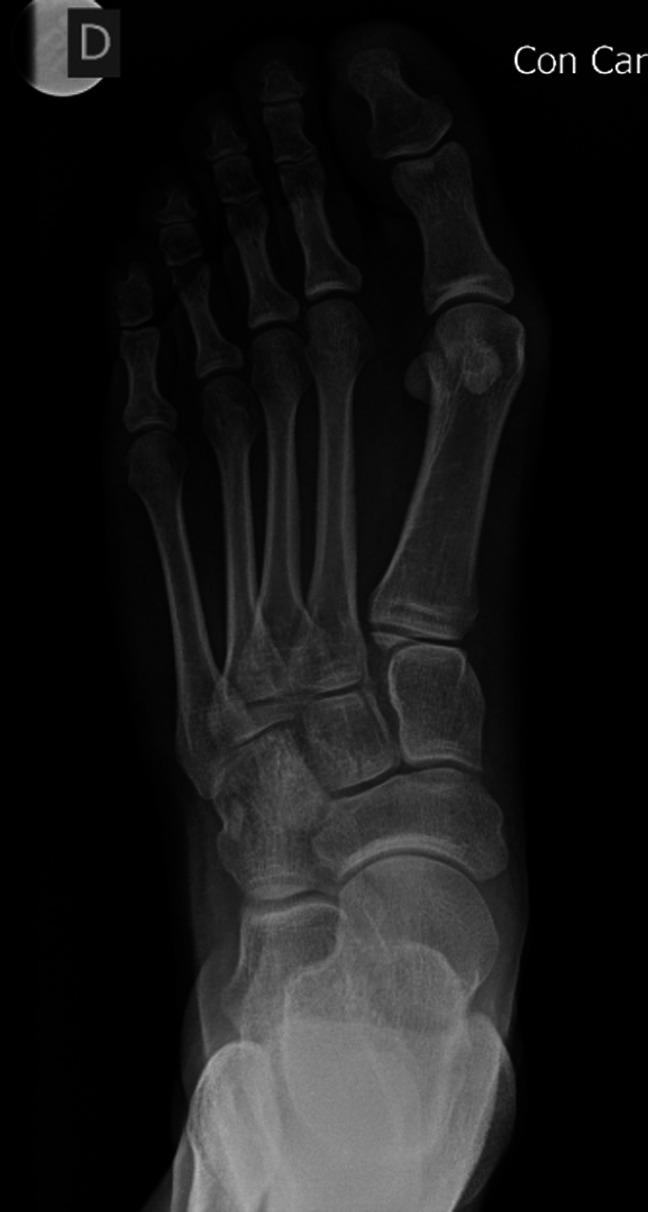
Radiograph demonstrating the anterioposterior section of a patient with hallux valgus. Note the apparent lateral sesamoid subluxation in relation to the metatarsal head.
